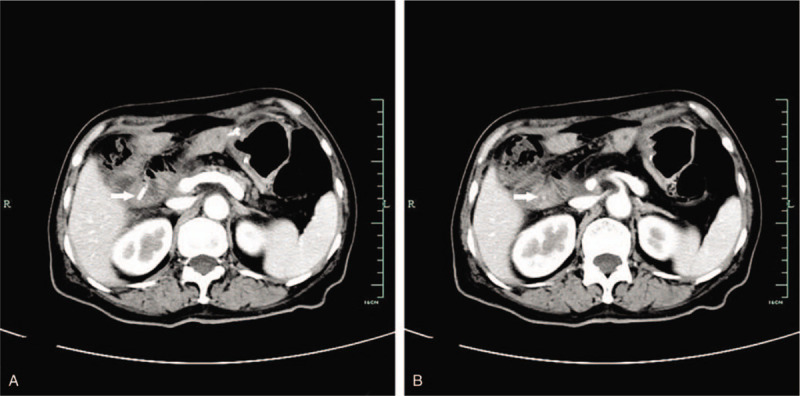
Abdominal enhanced computed tomography 6 months after surgery showed that the residual stent (arrow) remained inside the bowel lumen without other anomalies.
